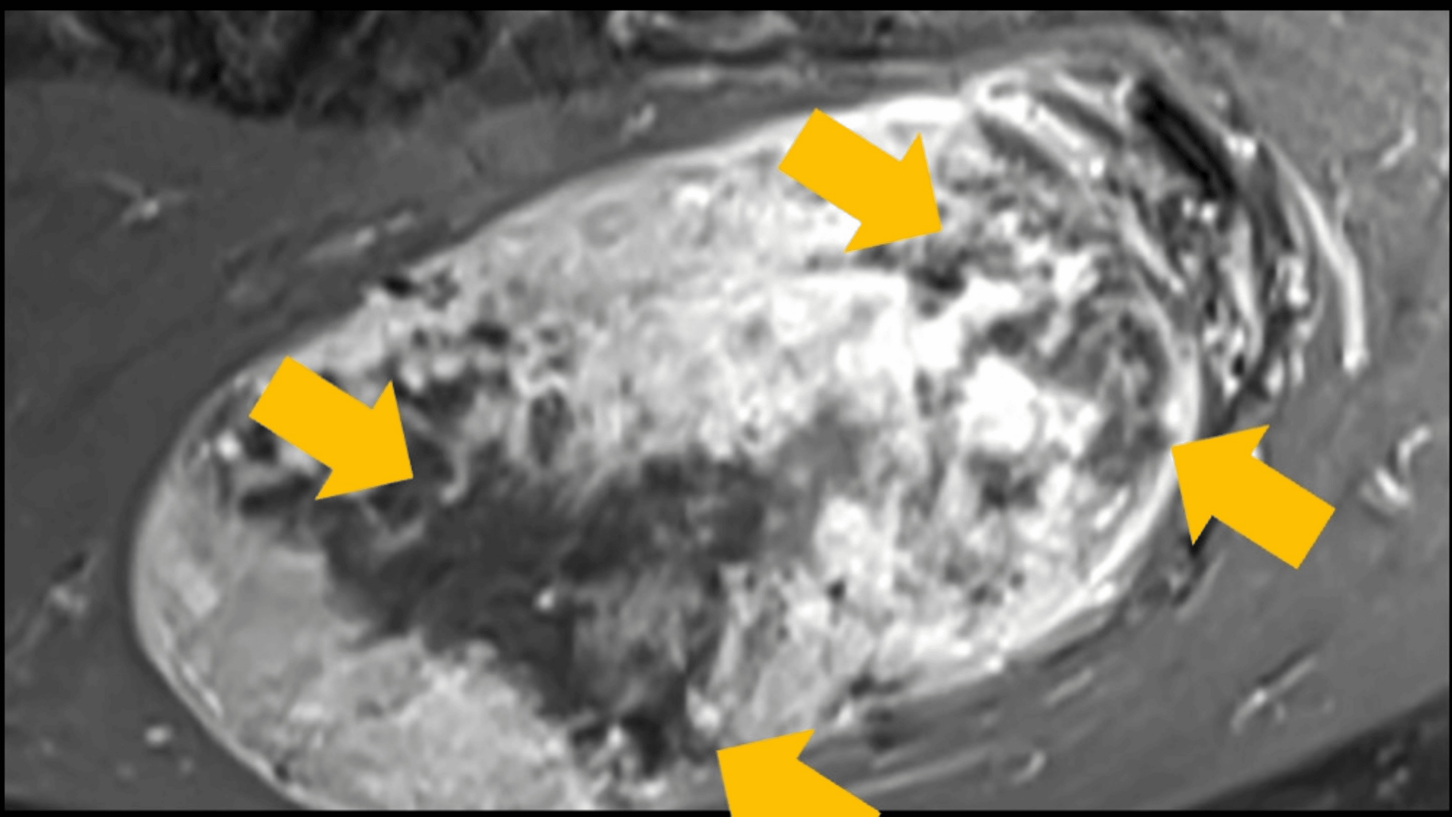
MRI of a large gluteal mass MRI displaying a large exophytic gluteal mass MRI: magnetic resonance imaging
